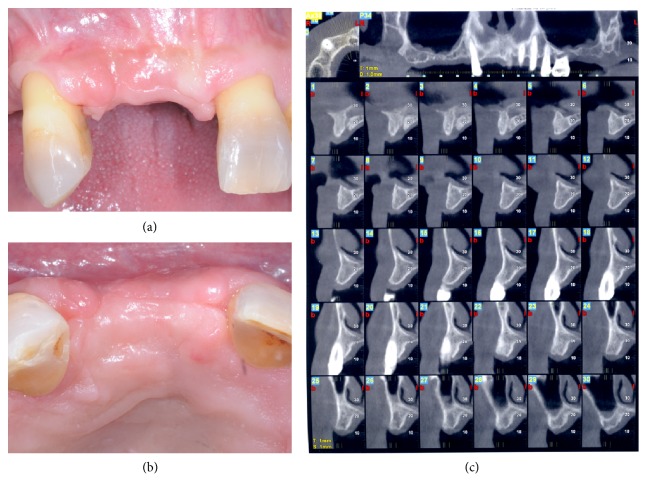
One year after the ridge reconstruction procedure ridge regeneration was achieved. Buccal view (a); occlusal view (b); cone-beam computed tomographic scan (c).
